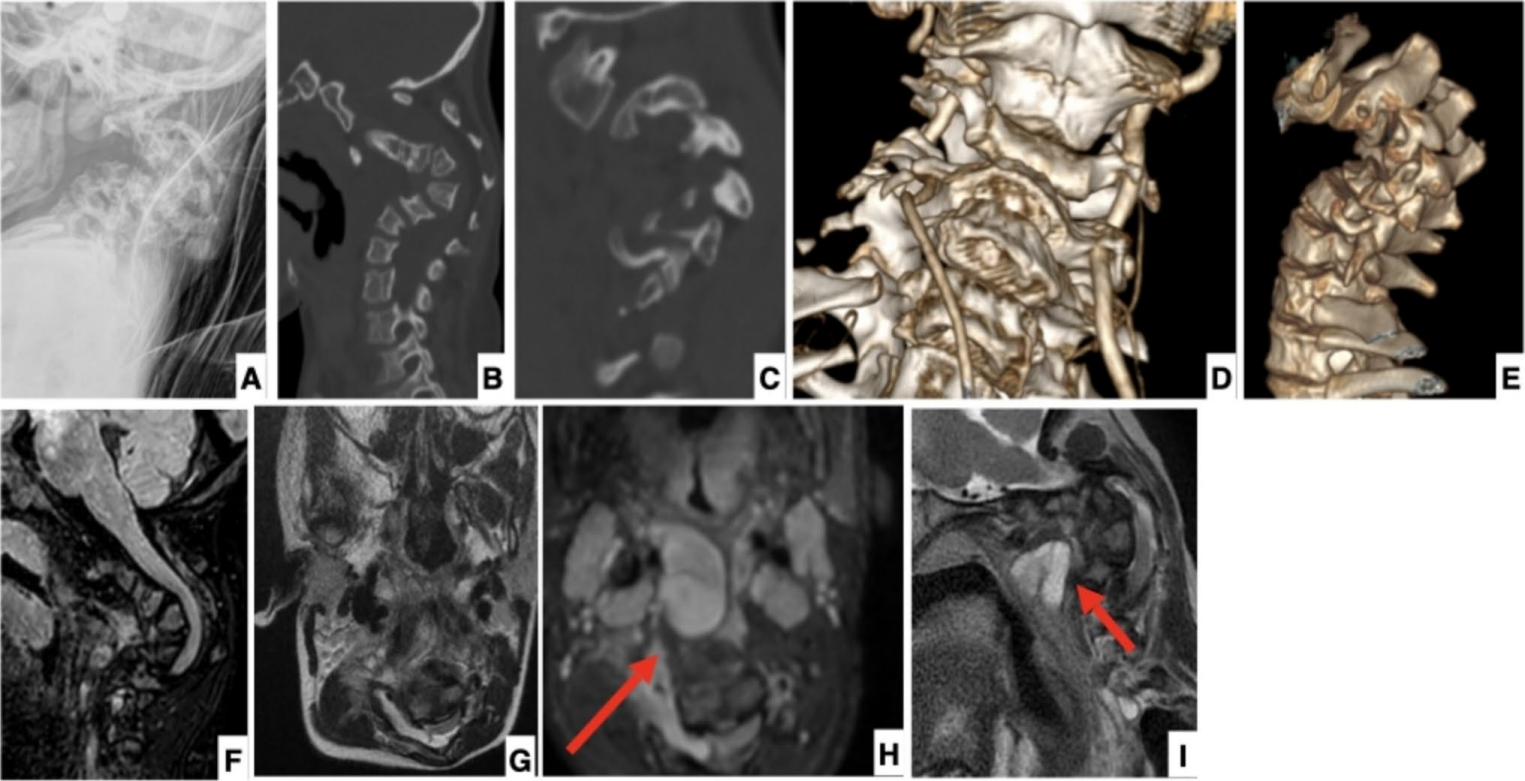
Imaging of the patient. A Pre-operative lateral view of the cervical spine. A severe kyphosis is demonstrated centered at C3. B CT scan reconstruction confirms the presence of dystrophic changes of the cervical vertebrae. C CT scan view of the right-sided facet joints shows dystrophic changes but no dislocation of the facets. D CT angiogram shows normal representation of the two vertebral arteries. E CT scan 3-D reconstruction of cervical spine deformity. F MRI scan shows tenting of the spinal cord around the kyphosis apex; the patient had no neurological deficits before surgery. G Axial image of the MRI confirms tenting and compression of the spinal cord at the apex of the kyphosis. H MRI axial image showing the extensive pre-vertebral neurofibromas (red arrow) encasing major blood vessels of the neck. The extensive neurofibromas present in the front of the spine made the anterior approach not feasible in this patient. I MRI sagittal view confirms the presence of extensive pre-vertebral neurofibromas (red arrow)
